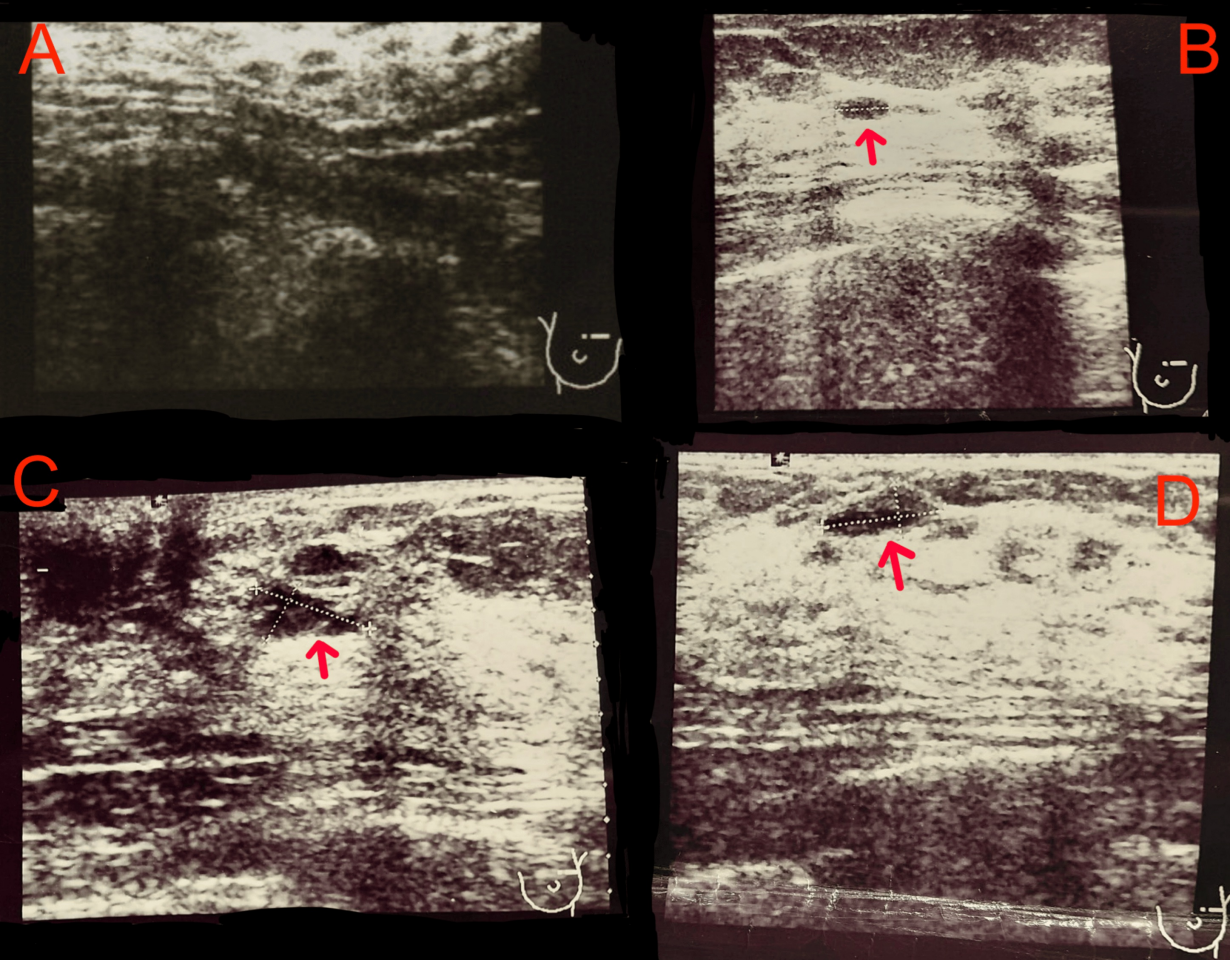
Breast ultrasound examination on March 13, 2018 A: Breast with predominantly glandular-adipose composition; B: Cyst measuring 5 mm in diameter (red arrow); C: Cluster of milk ducts measuring 7×4 mm (red arrow); D: Lesion of mixed echogenicity measuring 9×4 mm, suggestive of a mastopathic cluster (red arrow).
